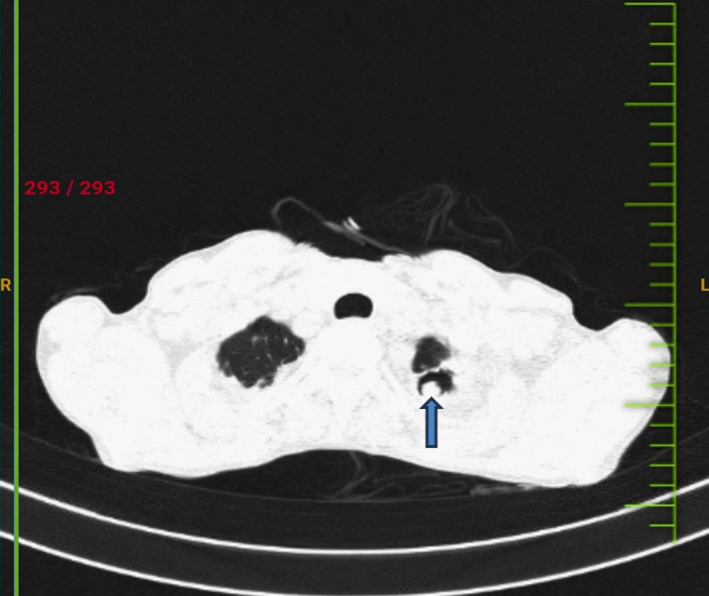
axial section of computed tomography of lung showing a cavity in left upper lobe (blue arrow) with a rounded mass in it with a surrounding crescent shaped air shadow (the Air crescent sign)
